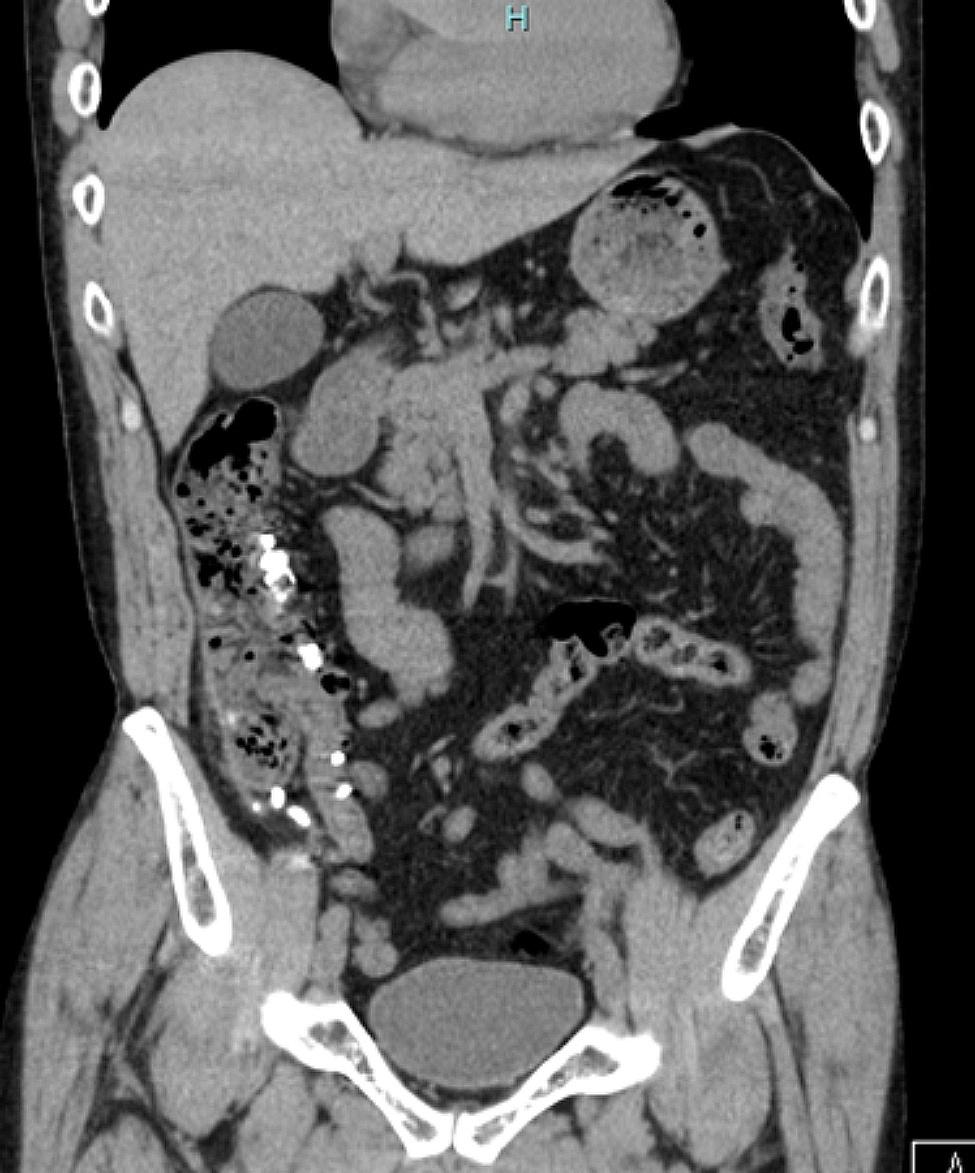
A non-contrast CT scan of the abdomen in case 1 CT shows multiple highly absorptive diverticula in the ascending colon, some with free air. CT, computed tomography
